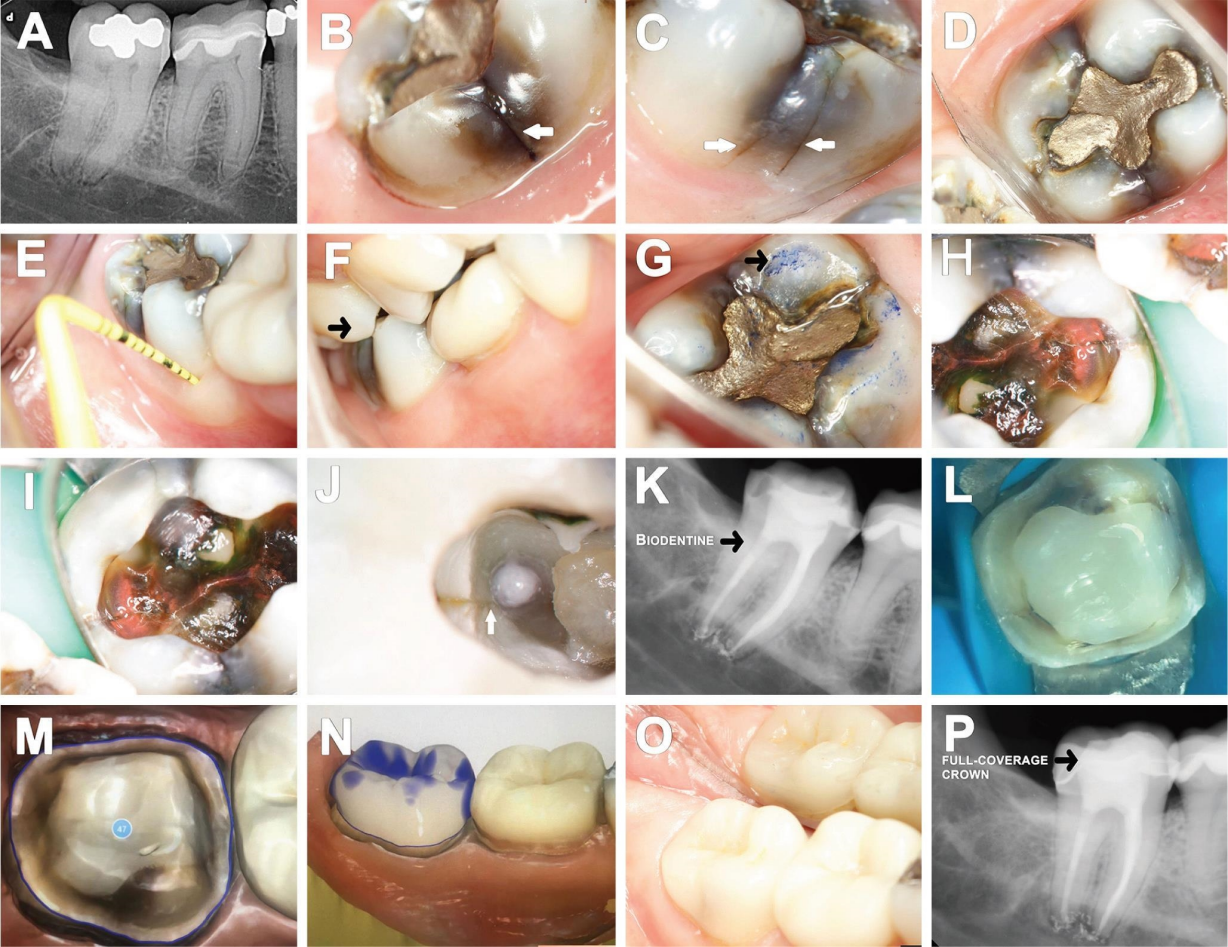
Initial digital radiography of tooth #31 shows symptomatic apical periodontitis (A); the presence of CLs in the lingual, buccal, mesial, and distal surfaces and class I amalgam restoration was identified under DOM (B–D); no periodontal probing depth was noticed (E); waste face and occlusal interferences were observed in the working movements (black arrows) (F, G); amalgam was removed, and CLs were assessed under DOM (H, I); internally, the crack line extended 2.0 mm beyond the level of the distal canal orifice (white arrow) (J); endodontic treatment was performed and Biodentine™ intraorifice barrier was placed 2.0 mm deepest the CL (black arrow) (K); preparation of full-crown coverage was performed and images from CEREC (Zurich, Switzerland) milling machine (L–N); bonded full-crown coverage in E-MAX (Ivoclar Vivadent, SP, Brazil) was fixed (O); final digital radiograph was taken after definitive restoration (P).
